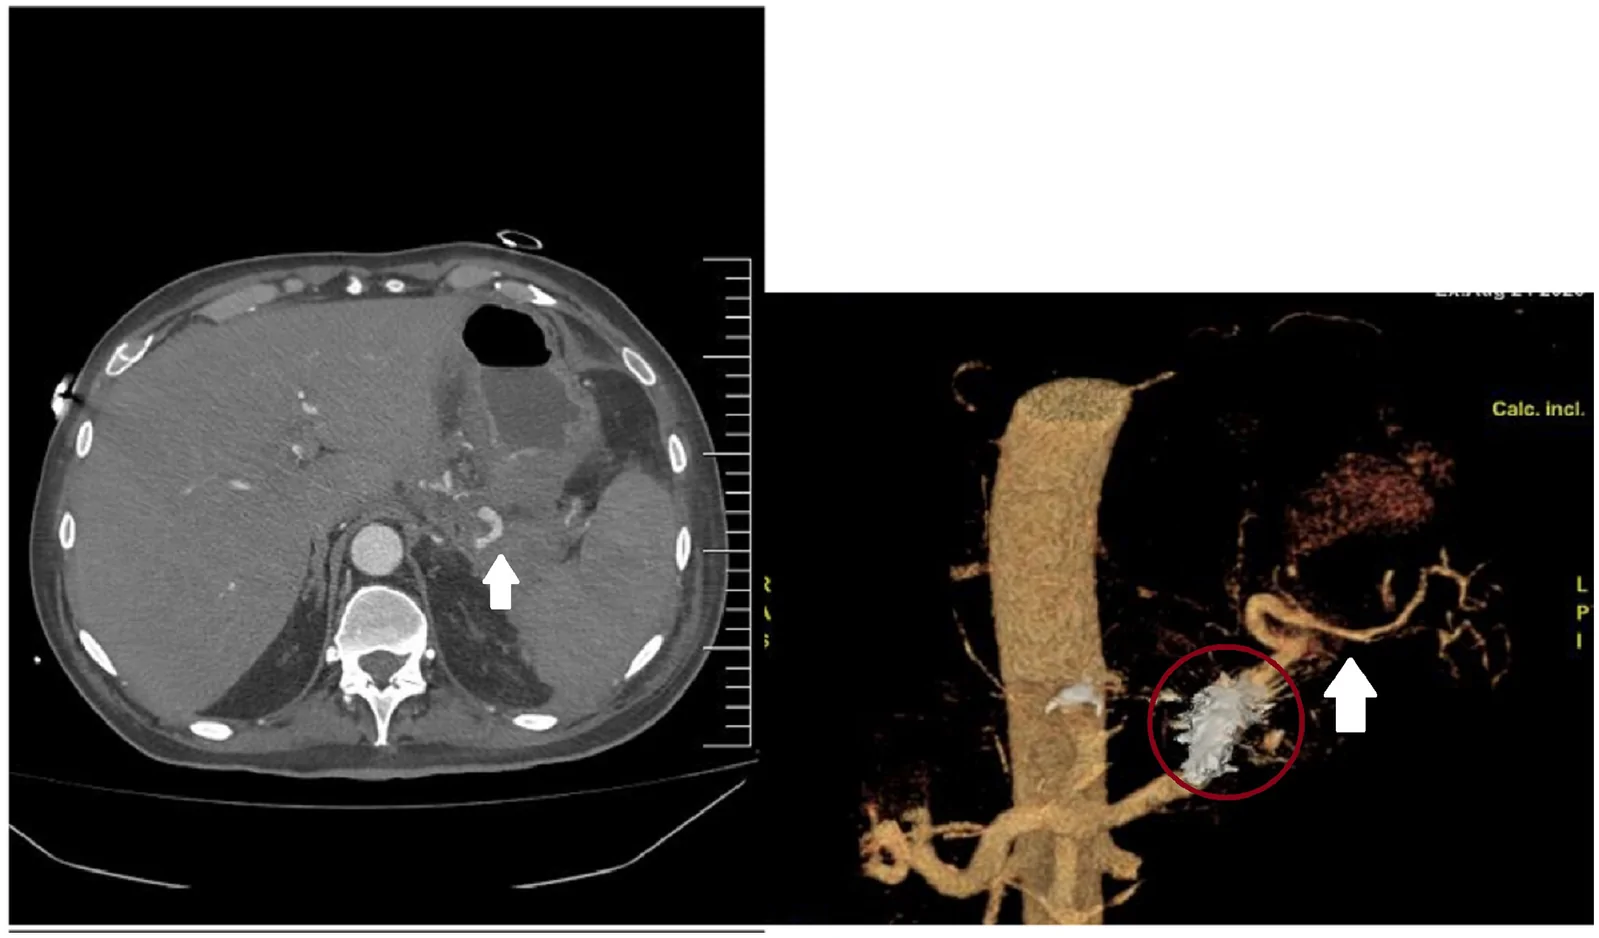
Postembolization multiphasic CT showing persistent hyperdense splenic foci and distal arterial flow beyond the embolization coils with arterial leak. Circle indicating coil embolization of the splenic artery; the arrow points to persistent arterial flow in the splenic artery despite proximal embolization.
